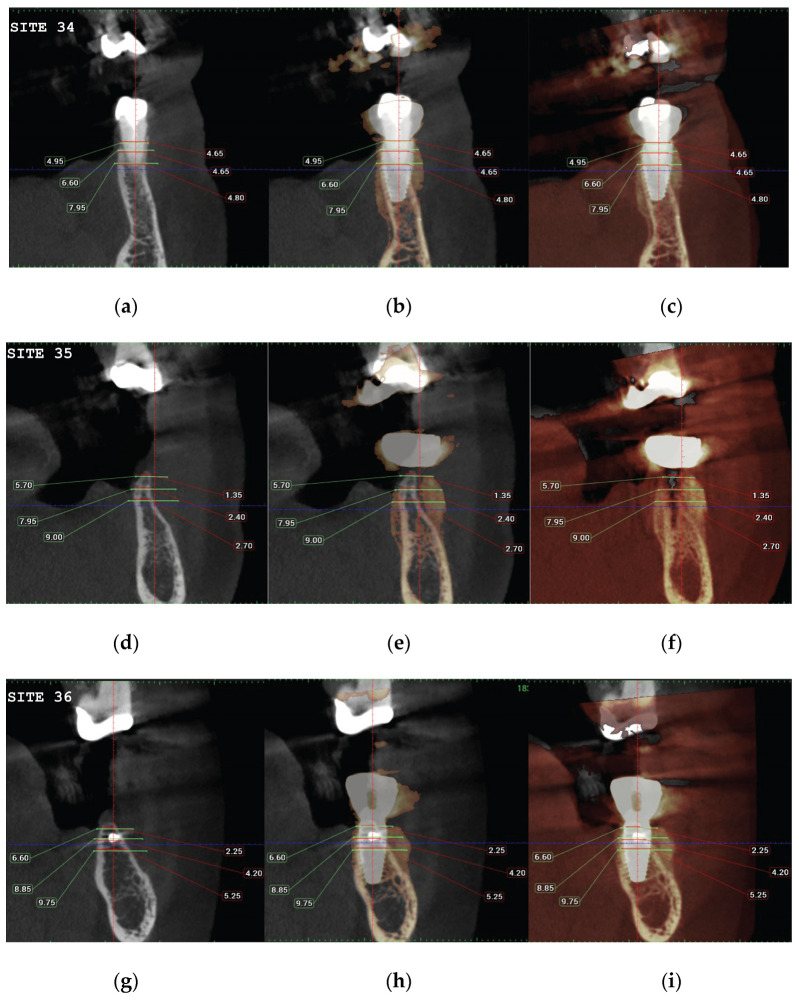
(a) The T1 CBCT image of site 34, (b) the superposed images T1-T2, (c) and the post-image T2, with measurements overlaid. (d) The T1 CBCT image of site 35, (e) the superposed images T1-T2, (f) and the post-image T2, with measurements overlaid. (g–i) Similar setup for the molar 36 site.
